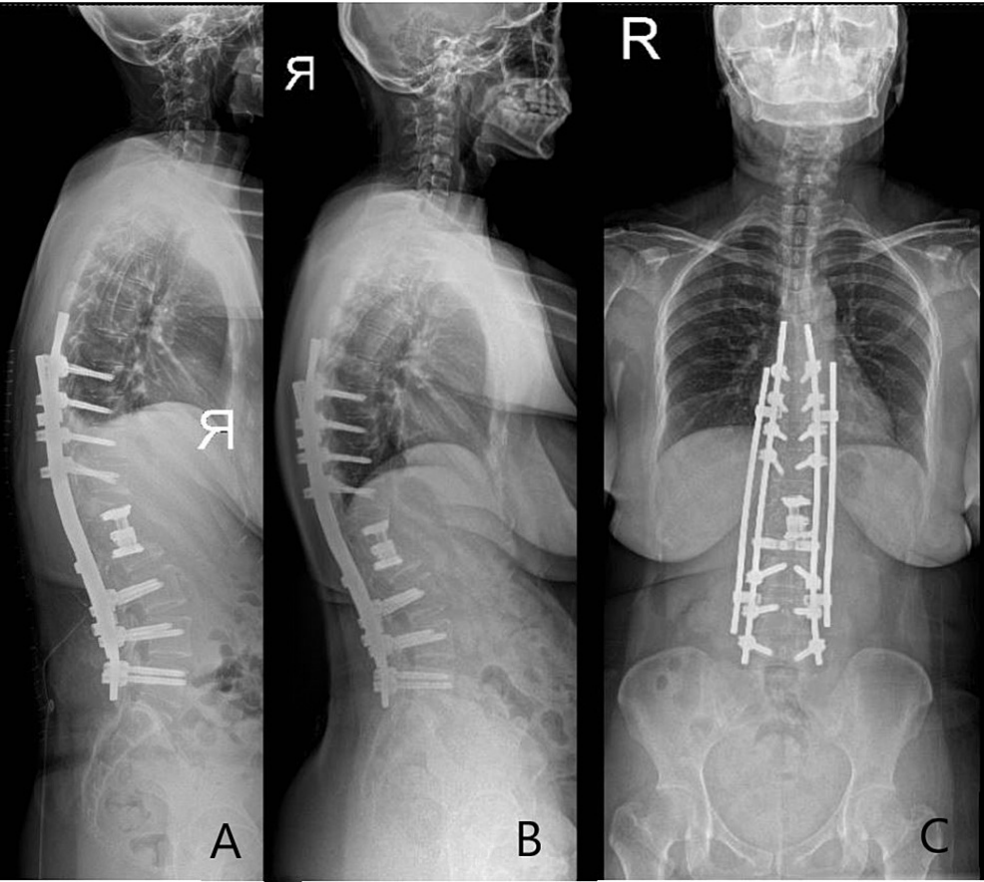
Immediate postoperative standing lateral whole-spine radiograph with posterior instrumentation and cage insertion (A). Three-year postoperative lateral (B), and anteroposterior (C) whole-spine radiographs.
